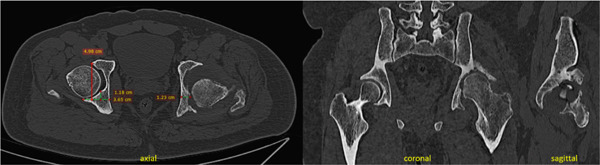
Case 3 preoperative computed tomography.
